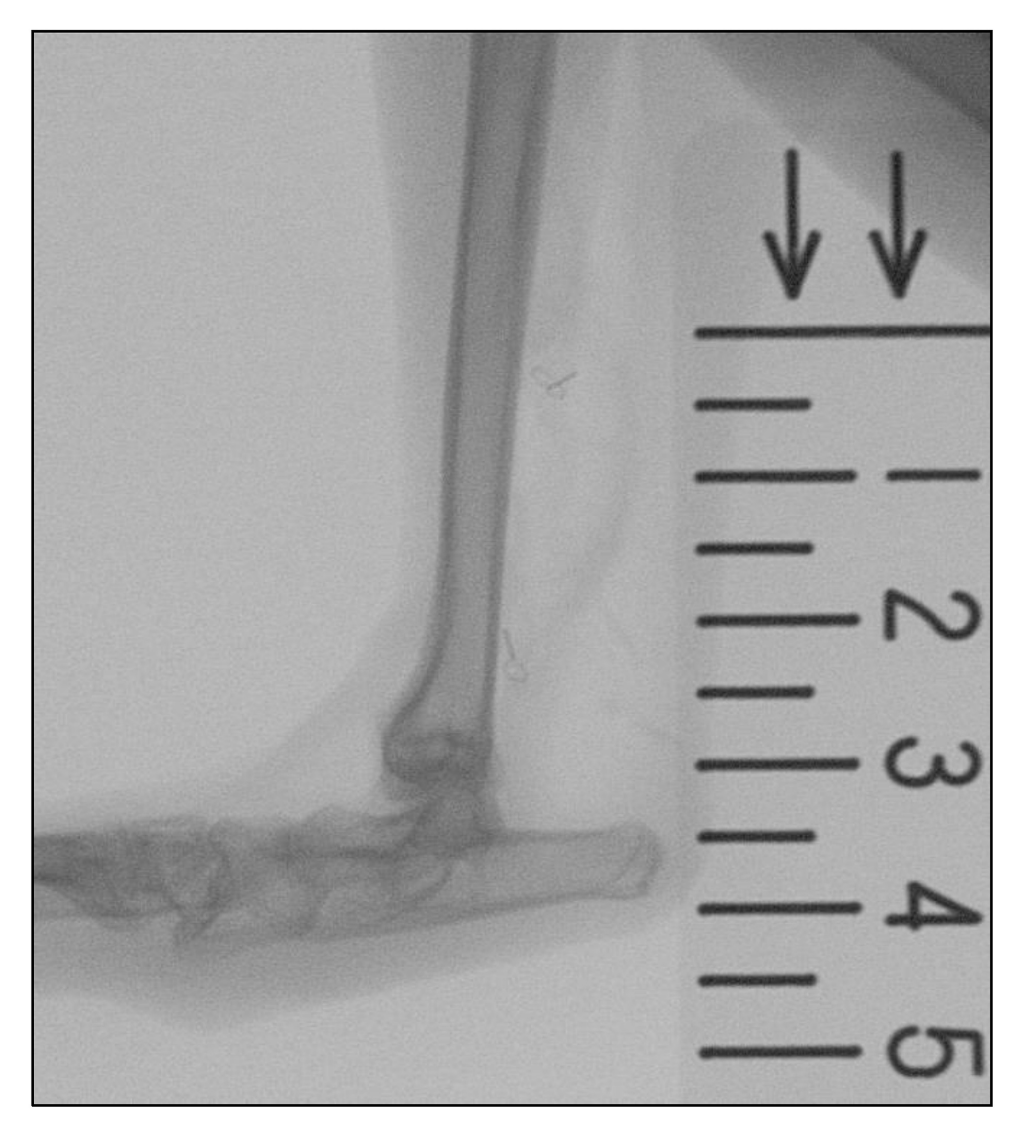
Radiograph of a treated ankle with radiopaque wires marking the ends of the incision
